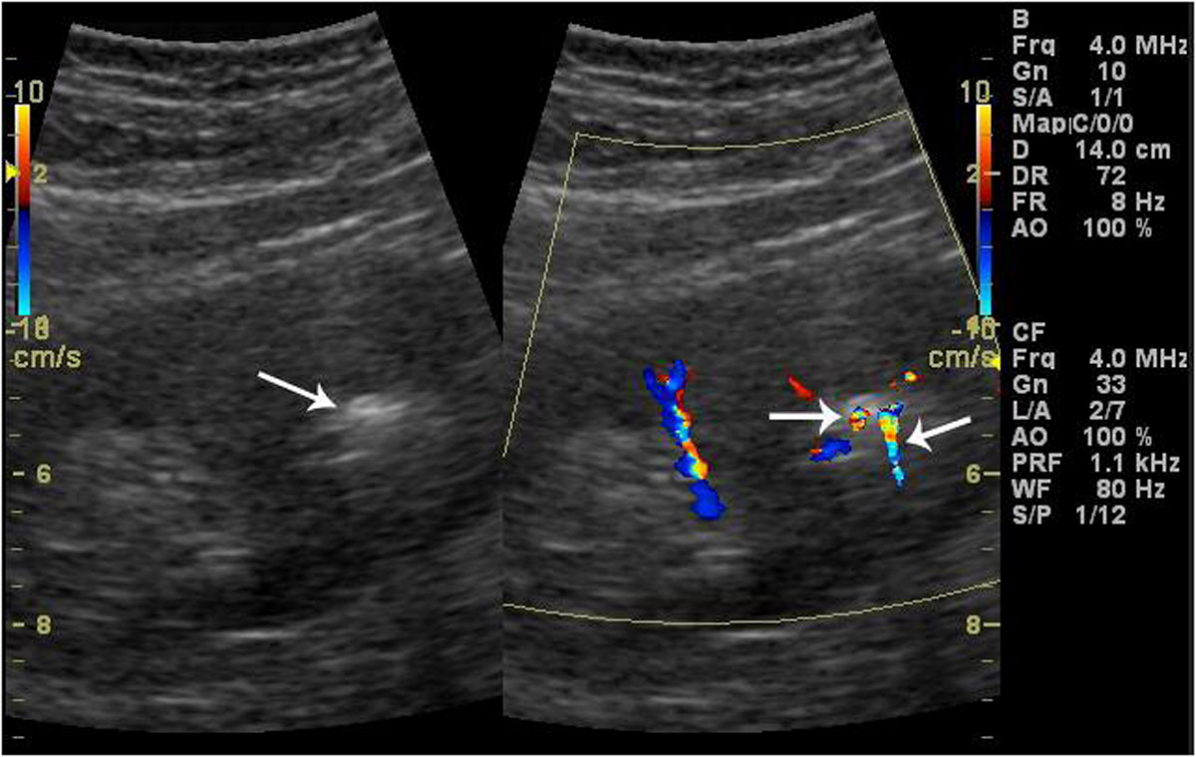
Two small renal stones with calyceal dilatation. Grey-scale sonogram shows only one hyperechoic spot with posterior acoustic shadowing. Color Doppler sonogram reveals two twinkling signs.
